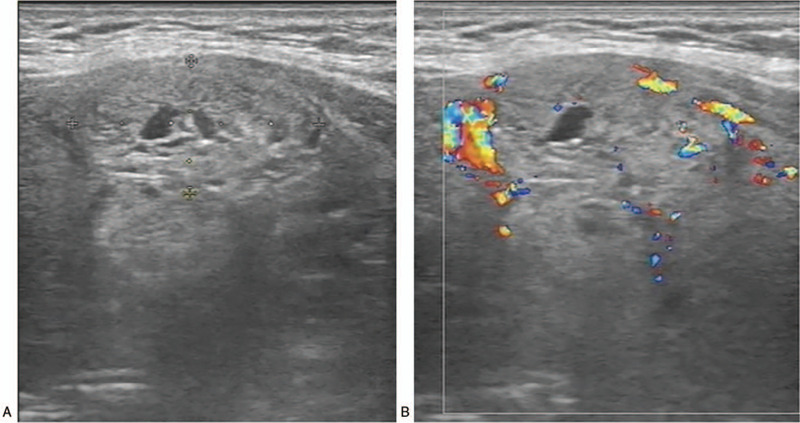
Ultrasound images of the patient in 2015. (A) A 2-dimensional image showing the size of the nodule of about 2.5 × 1.3 cm, with small patchy fluid sonolucent area was found in the lesion. (B) CDFI showed circular blood flow signals around the nodule and poor blood flow signals inside the nodule.
